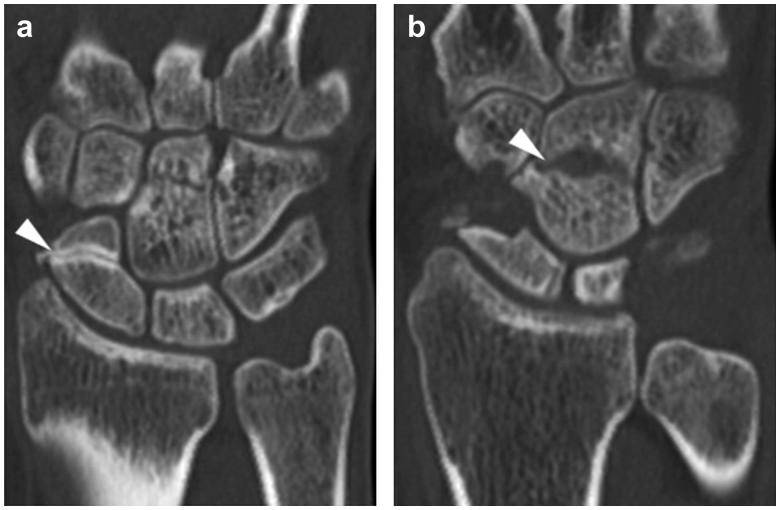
Computed tomography (CT) scan of the hand. Sagittal CT image of bone separation with osteosclerosis in the distal part of the scaphoid bone (a), and a radiolucent line in the central area of the capitate bone (b).
